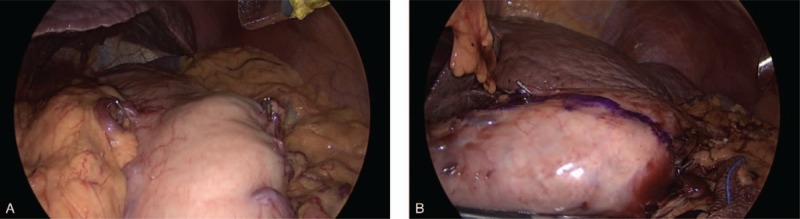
Intraoperative localization procedure. Two metallic clips were applied to the external surface of the stomach (A), Thereafter, the transection line was drawn using gentian violet based on the intraabdominal radiography (B).
